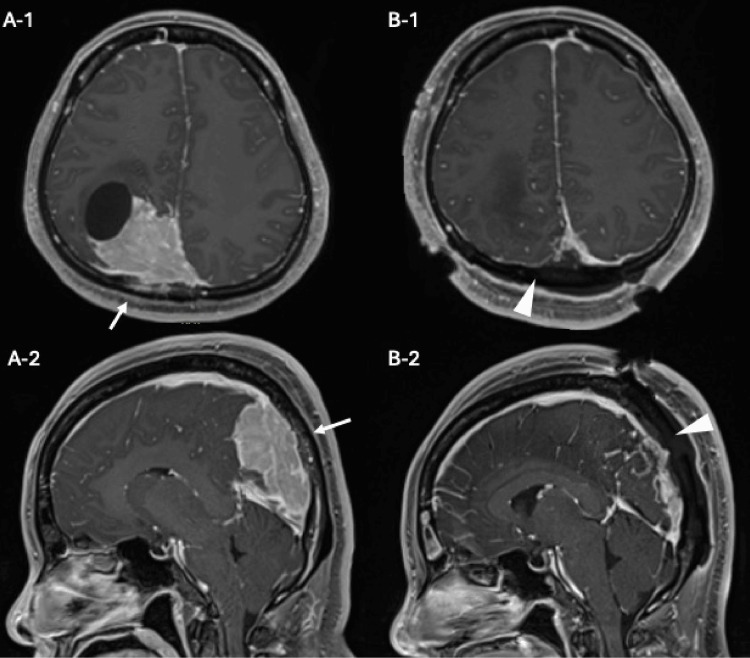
Contrast-enhanced MRI findings (A-1) Axial and (A-2) sagittal contrast-enhanced MRI images reveal a cystic tumor located near the sigmoid sinus in the parietal region, demonstrating uniform enhancement (white arrow). The tumor is observed invading the sigmoid sinus. (B-1) Axial and (B-2) sagittal contrast-enhanced MRI images post-craniotomy show successful removal of the tumor, with no visible brain damage (white arrow head).
